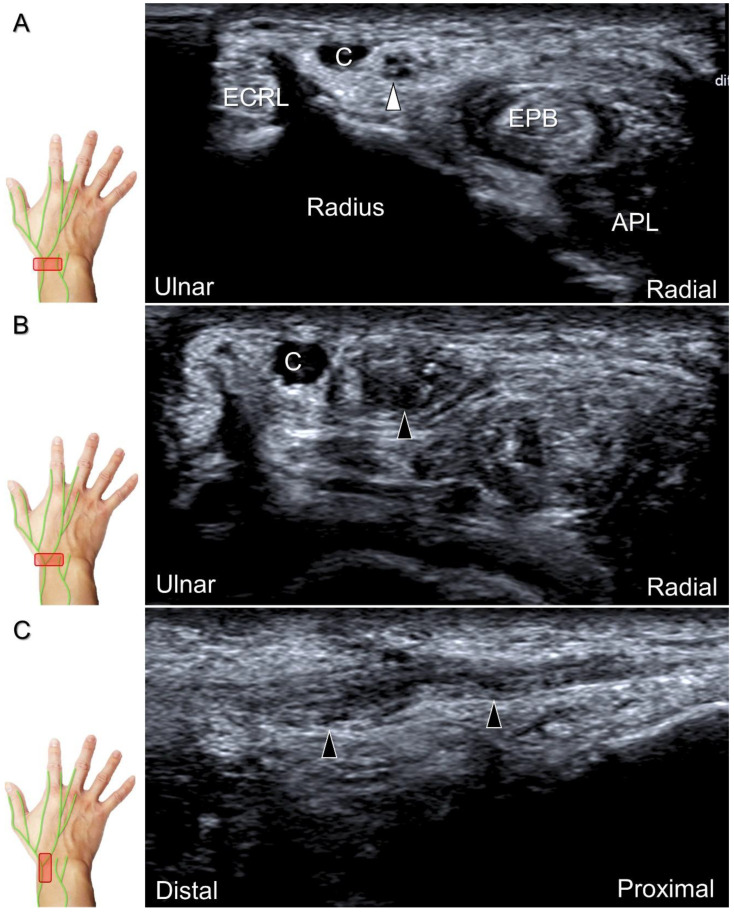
Sonographic images depict the location of a neuroma of the superficial radial nerve. The normal/proximal segment (A) and the neuroma in short-axis (B) and long-axis (C) views are seen. White arrowhead: superficial radial nerve; black arrowheads: neuroma; APL: abductor pollicis longus tendon; EPB: extensor pollicis brevis tendon; ECRL: extensor carpi radialis longus tendon; C: cephalic vein.
